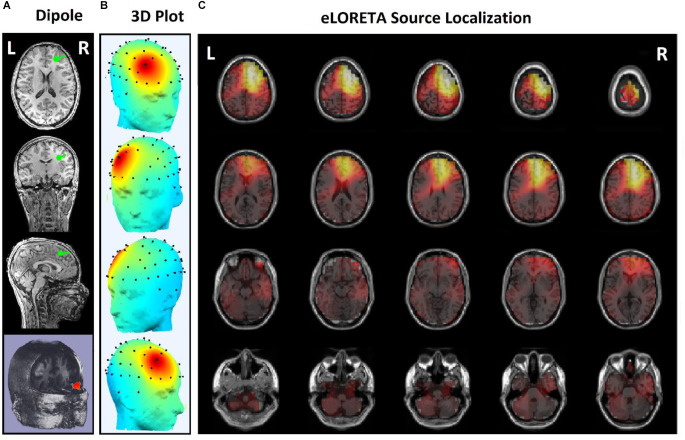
Source localization of rTMS stimulation foci in right prefrontal cortex (R-DLPFC) for a non-responder subject. (A) Dipole coordinates of the identified focus in right side on a default MRI. (B) Position of the foci identified by ICA on the surface of the skull. (C) Source localization in right DLPFC by eLORETA.
